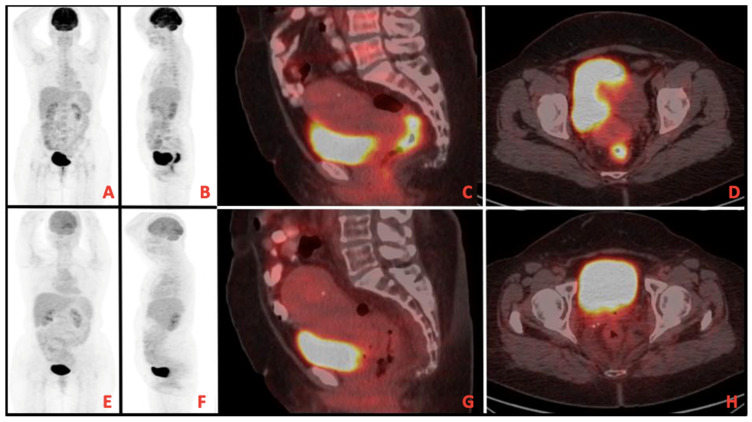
(A–D) Pretreatment imaging, (E–H) posttreatment imaging on positron emission tomography/computed tomography (PET/CT).
